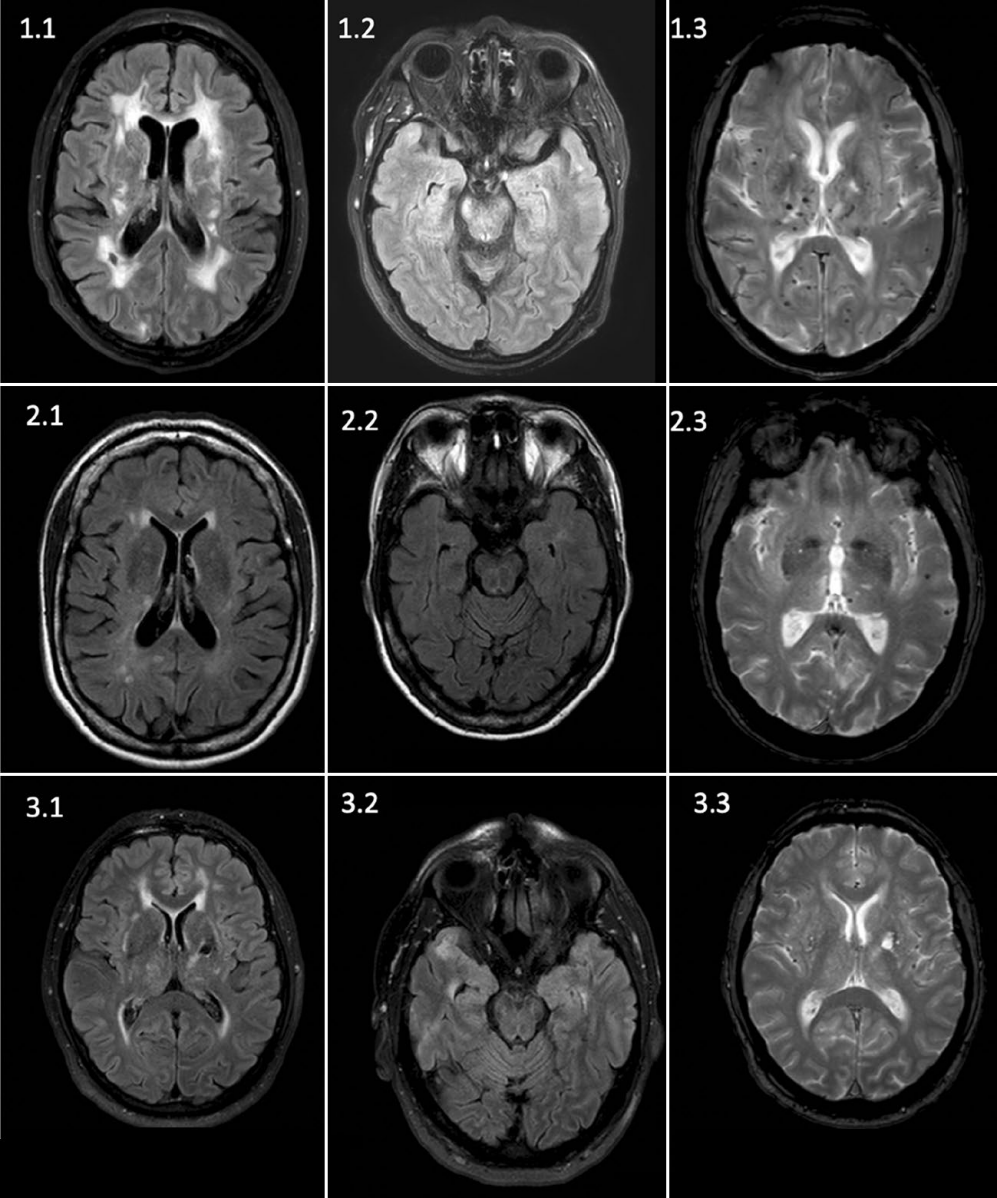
MRI images of the three CADASIL patients identified in our cohort. Patient 1 refers to the known CADASIL disease. 1.1: FLAIR sequence showing lacunar defects and extensive confluent white matter lesions, 1.2: FLAIR sequence, white matter lesions without emphasize on temporopolar region, 1.3: SWI sequence showing extensive microbleeds both thalamic and cortical. Patient 2 refers to the clinically suspected patient. 2.1: FLAIR sequence with extensive white matter lesions. 2.2: FLAIR Sequence, no emphasize on temporopolar, 2.3: T2* (“heme”) sequence showing supratentorial cortical microbleeds as well as thalamic microbleeds. Patient 3 refers to the clinically not suspected patient. 3.1 FLAIR sequence showing temporal white matter lesions and a lacunar defect in the thalamus, 3.2: FLAIR sequence, temporal white matter lesions, 3.3: T2* sequence, no microbleeds were shown
